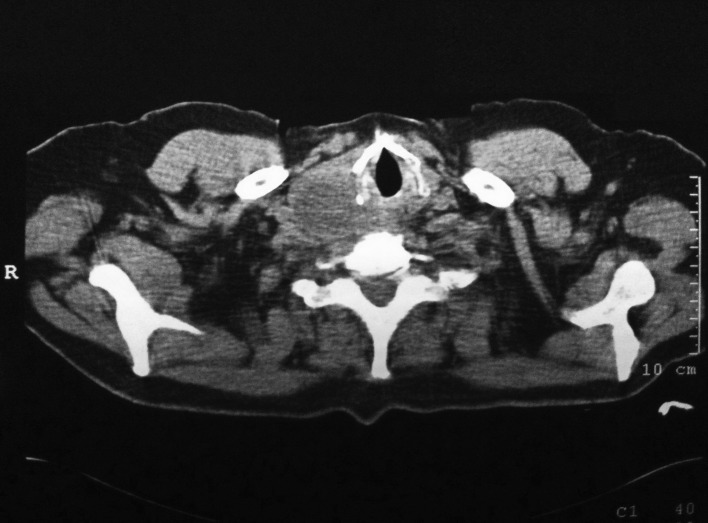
Computed tomography scan depicting a nodular lesion of the right lobe of the thyroid gland.
